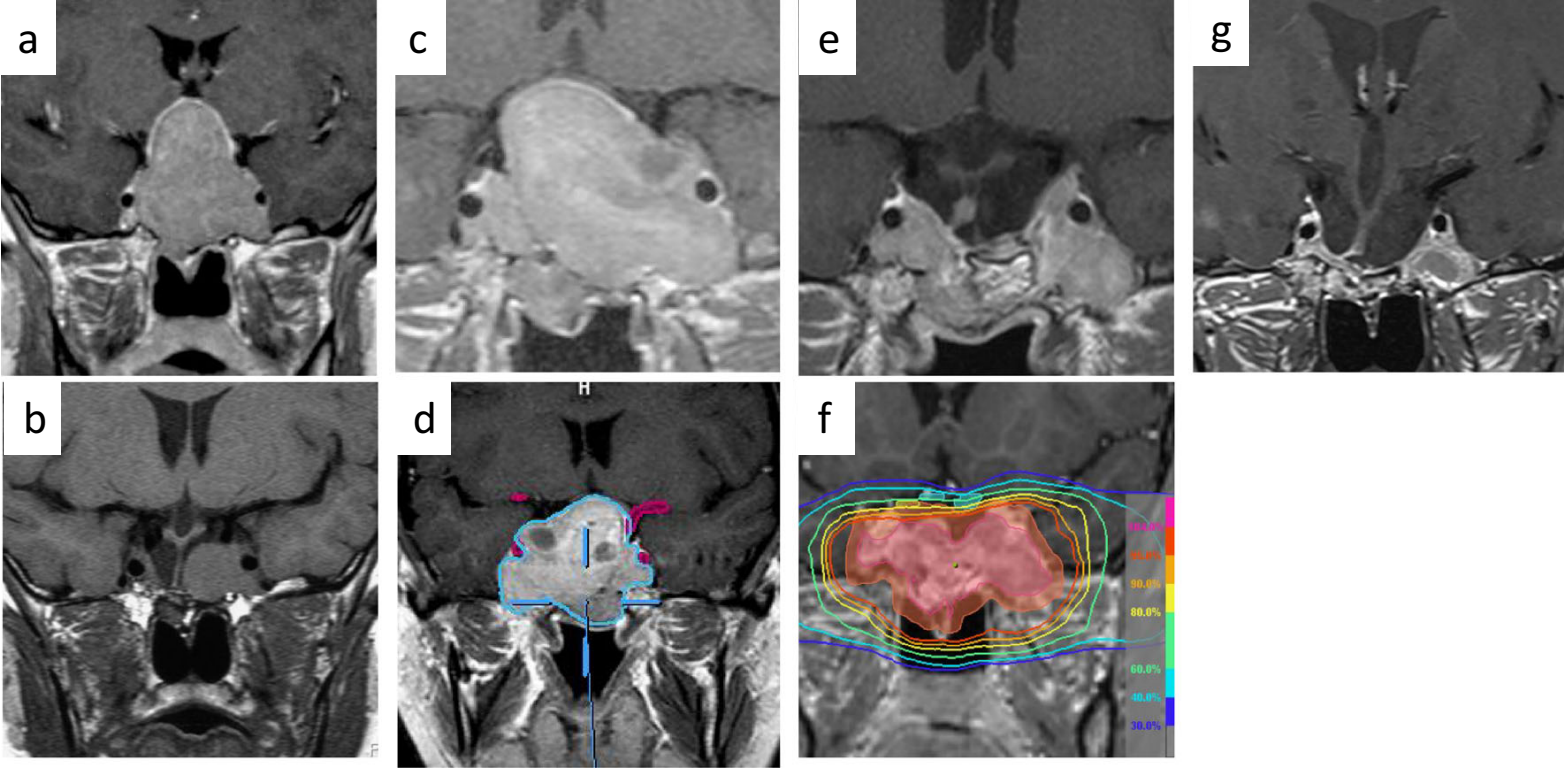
Rapid progression of a non-functioning pituitary adenoma in a 30-year-old female patient, as depicted on T1-weghted coronal MR images: a on presentation b delayed postoperative imaging 3 months after transsphenoidal surgery c follow-up investigation after 2 years d superimposing tumor structures onto operative field during repeat transsphenoidal surgery e delayed postoperative imaging after repeat surgery f RT-planning of residual tumor g most recent follow-up 5 years after conformal fractionated irradiation
